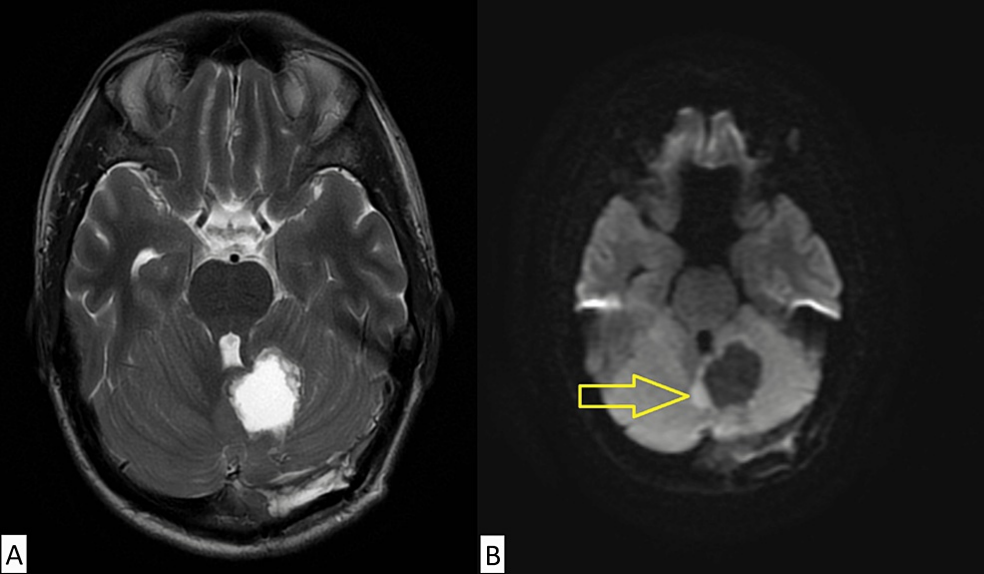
Postoperative MRI: (A) T2-weighted and (B) diffusion-weighted axial images demonstrating residual tumor in the anterior and medial resection cavity. MRI, magnetic resonance imaging
